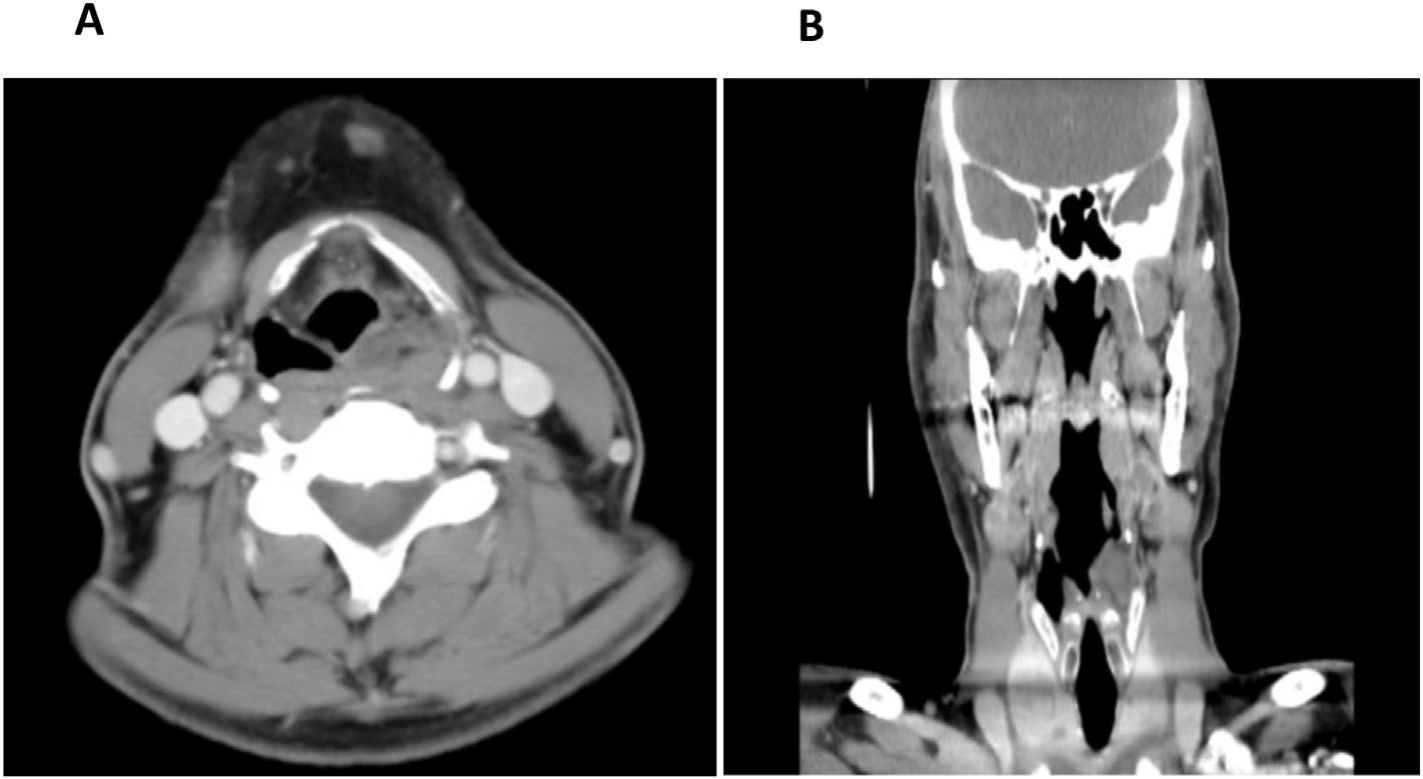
Computed tomography scan with contrast, showing obliteration of the piriform sinus on the left side with a faintly enhanced heterogeneous soft tissue lesion.
